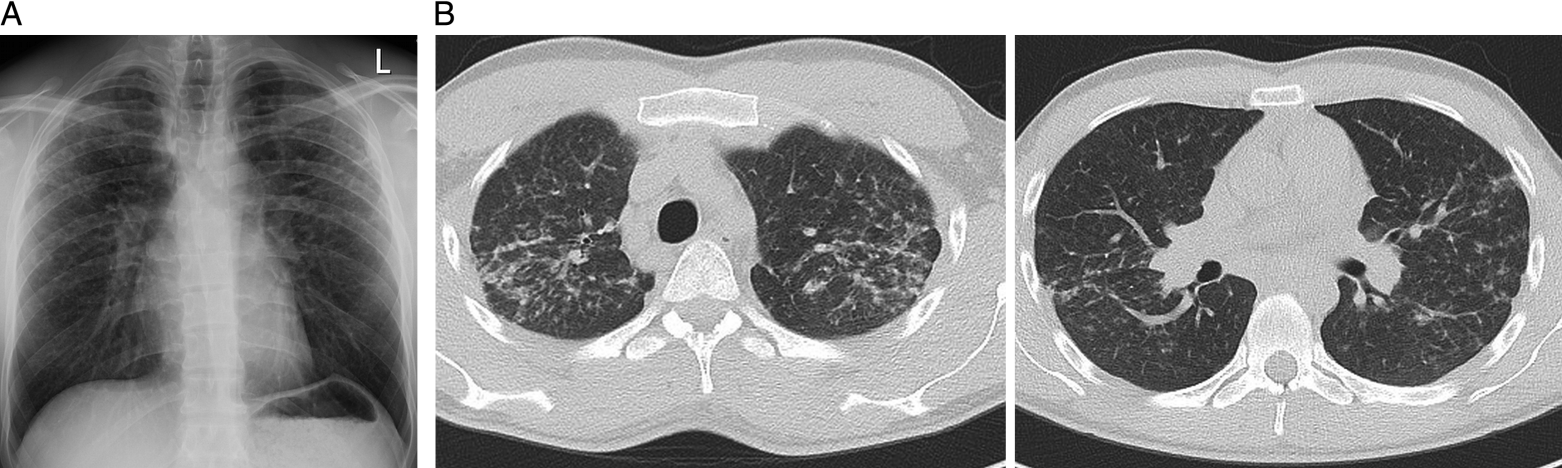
(A) Chest X‐ray showing bilateral upper lobe predominant infiltrates and (B) high‐resolution computed tomography scans demonstrating bilateral upper lobe reticulonodular infiltrates with fibrosis.
